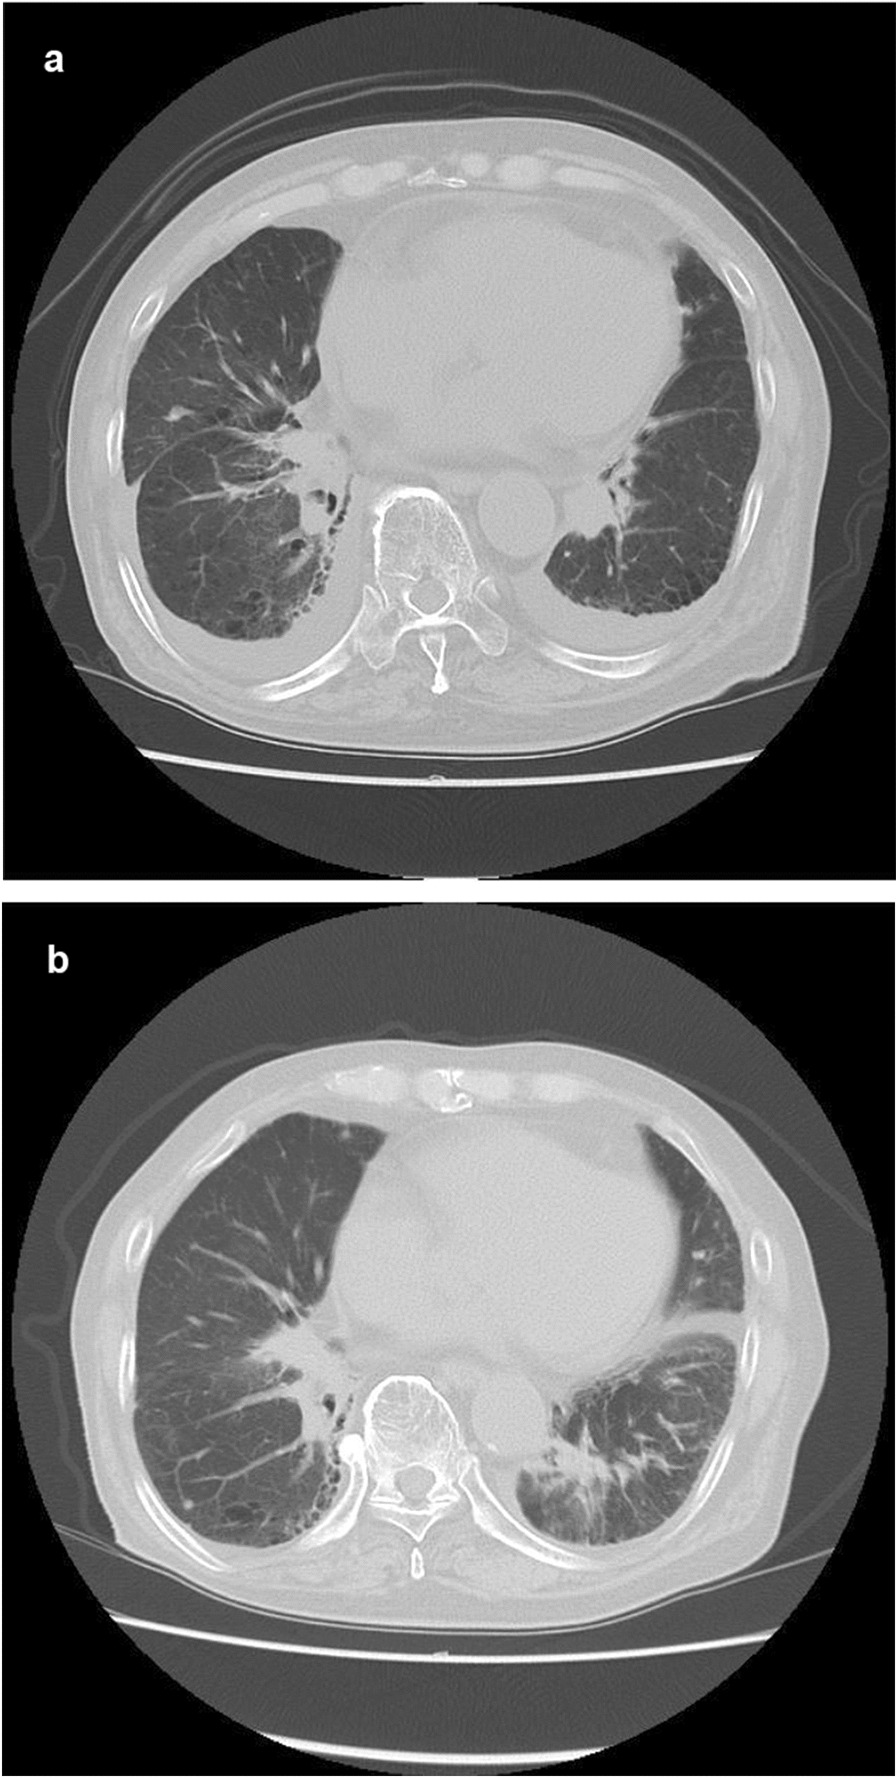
Computed tomography at a 2 months after the second stereotactic body radiation therapy and b 8 months after the second stereotactic body radiation therapy
